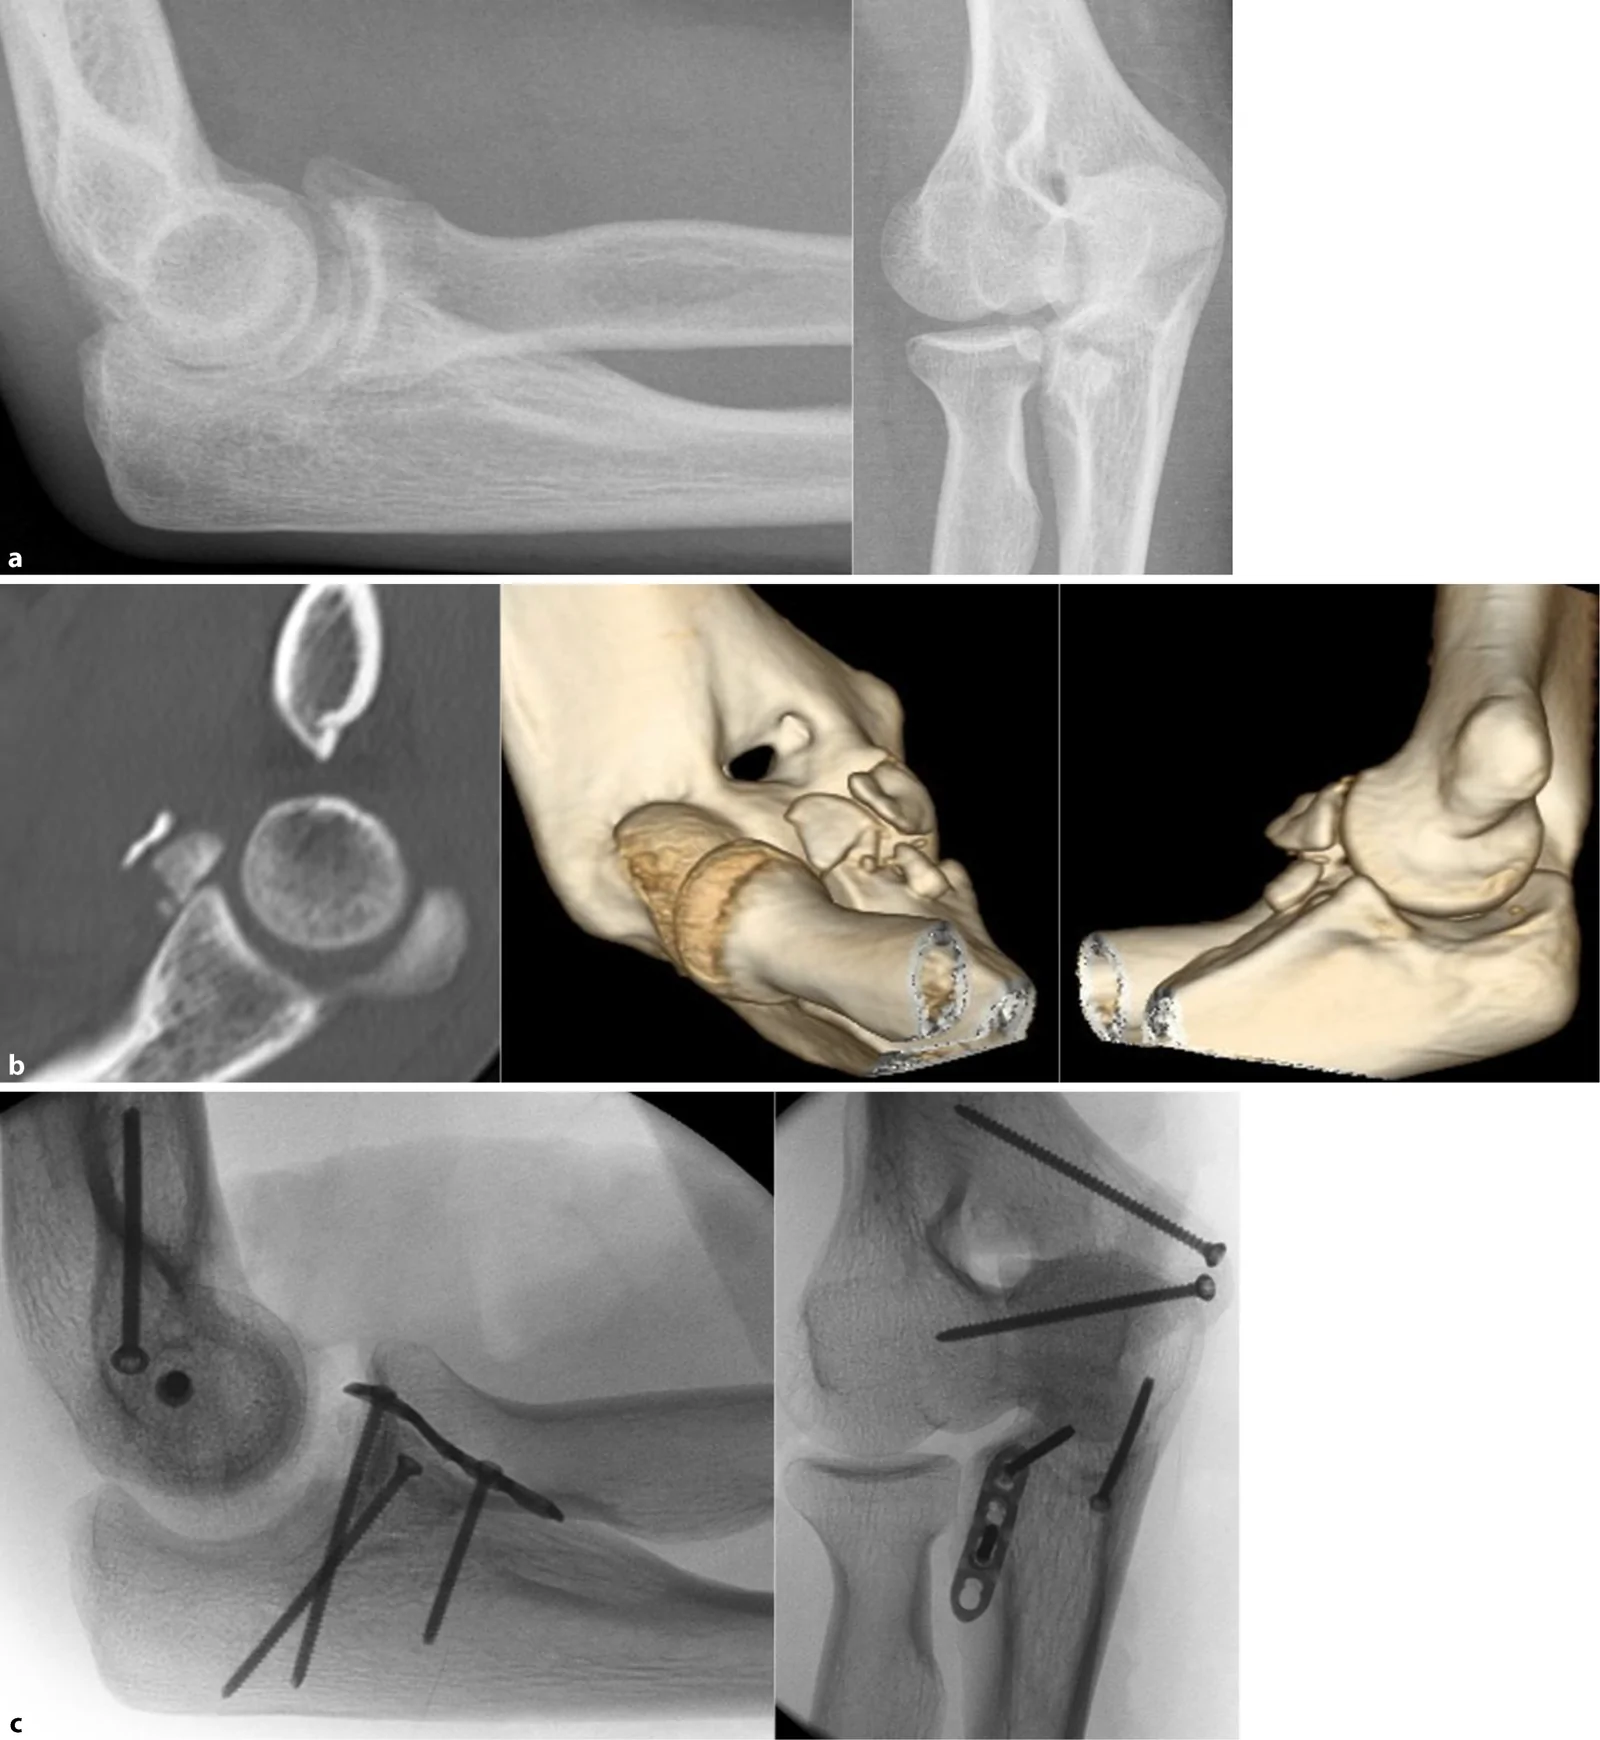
Radiographs (a) and computed tomography (b) demonstrate an O’Driscoll anteromedial type 2 fracture of the anteromedial rim and tip of the coronoid resulting in varus posteromedial rotatory instability. The fracture underwent fixation with a 2.0 mm buttress plate and an independent screw (c)
